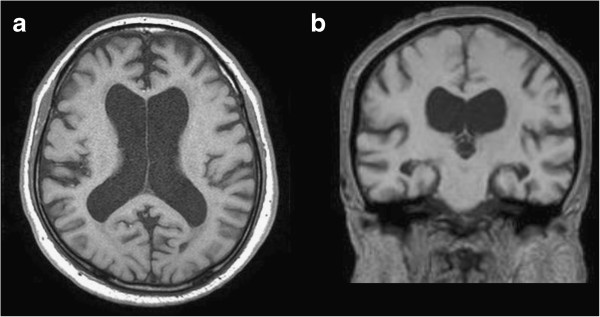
Magnetic resonance imaging scans of our patient. (a) Axial image showing lateral ventricular enlargement. (b) Coronal image showing narrowed subarachnoid spaces at the high convexity without severe cortical atrophy.
